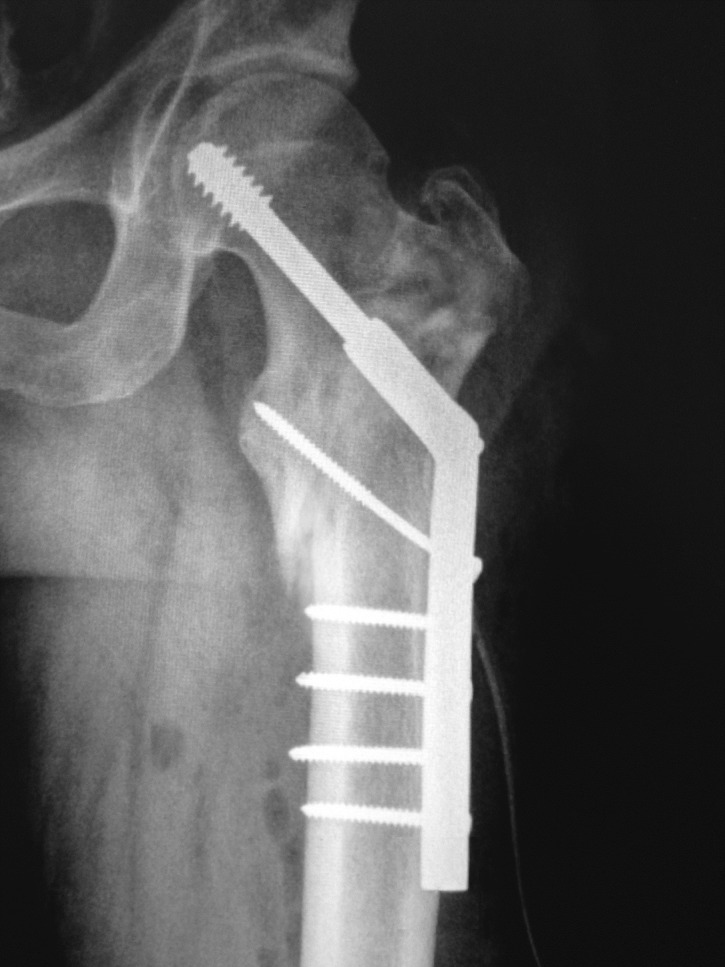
6 weeks postoperative antero-posterior X-ray view of the left hip
